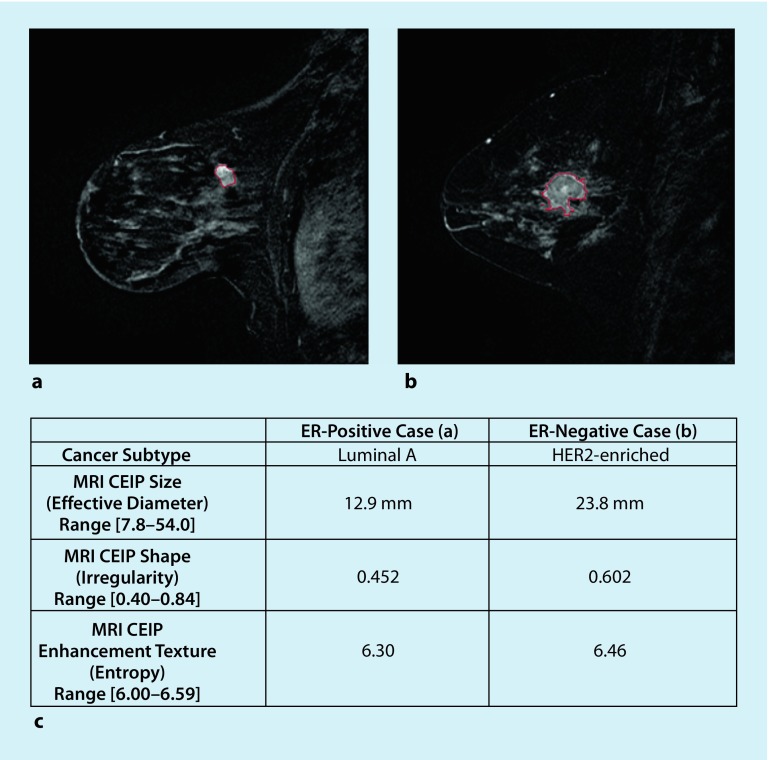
Example cases including segmentation outlines obtained from the computer segmentation method. a ER-positive example; b ER-negative example; c CEIP values (and ranges) for size, irregularity, and enhancement texture of two example cases. *CEIP* computer-extracted image phenotypes, *ER* estrogen receptor, *HER2* human epidermal growth factor receptor 2, *MRI* magnetic resonance imaging. (Reprinted with no modifications under a creative common license (https://creativecommons.org/licenses/by/4.0/) from [29])
